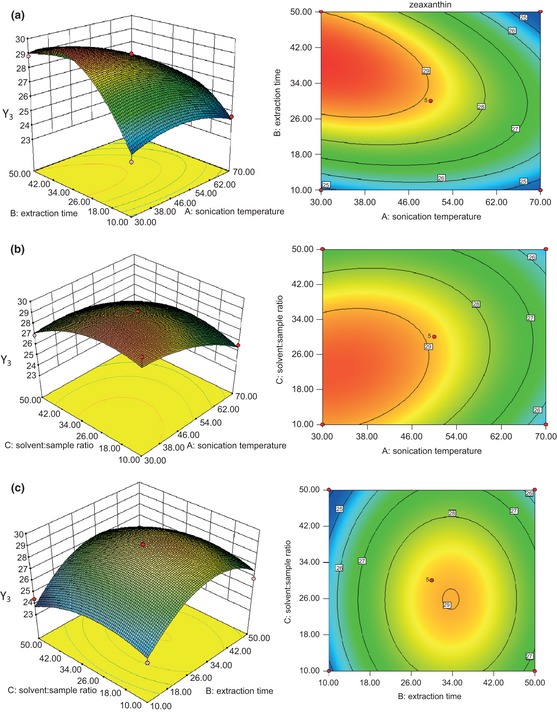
RSM contour and 3D surface plots of the effects of UAE conditions on zeaxanthin concentration. (a) Solvent:sample ratio (30:1); (b) time (30 min); (c) temperature (50°C). RSM, response surface methodology; 3D, three‐dimensional; UAE, ultrasonic‐assisted extraction.
